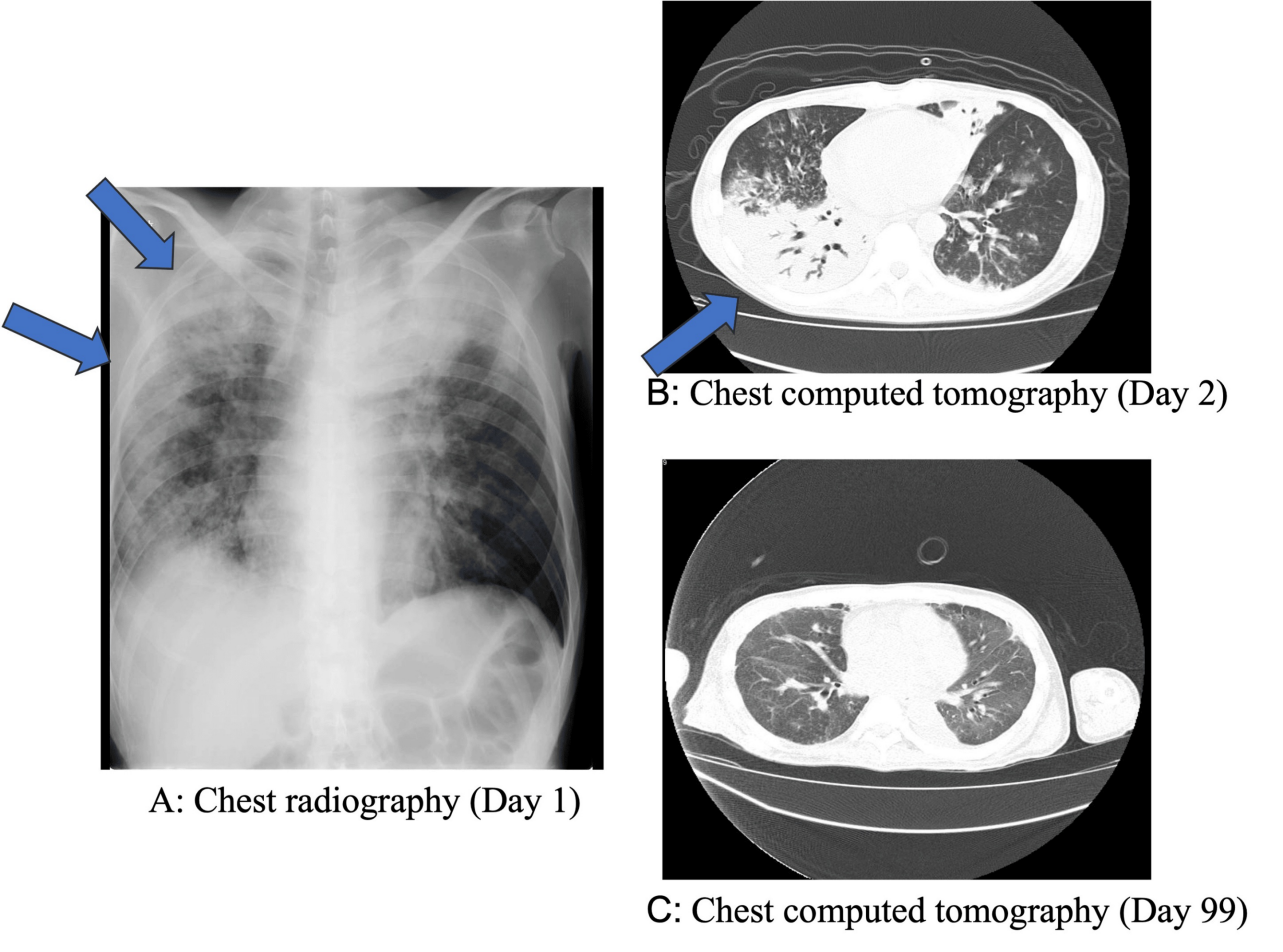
Chest radiography and computed tomography (Case 2) An infiltrative shadow (blue arrow) with bronchial translucency is present mainly in the dorsal right lung field on days 1 and 2.
